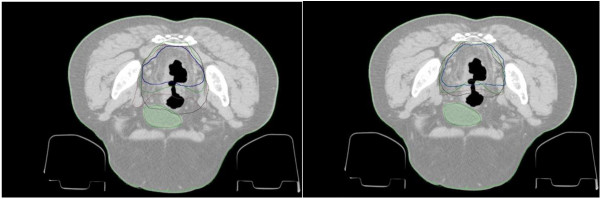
Four different contours of CTVs superimposed on a axial section of a CT image pre (left) and post (right) the introduction of guidelines.
